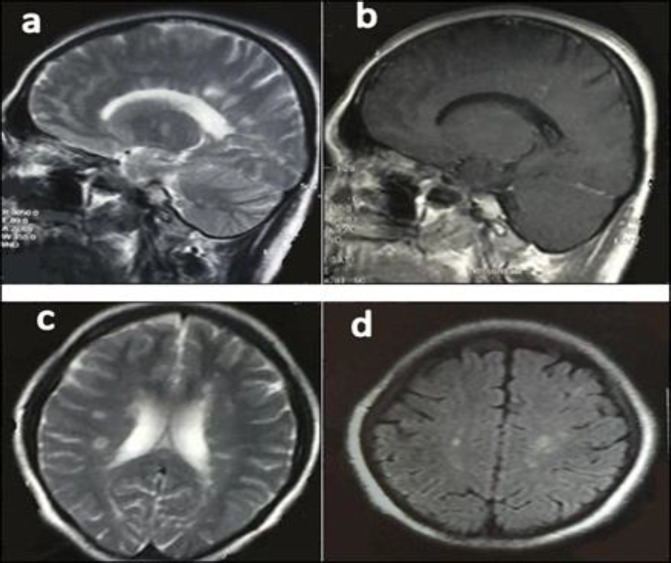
a) Sagittal T2 MRI, multiple hyperintense lesions involving periventricular white matter thalamus and cerebellum; b) Sagittal T1-weighted MRI showed enhancement of one periventricular lesion; c) and d) Axial T2 and FLAIR MRI, respectively; demonstrating multiple periventricular, centrum semioval, and juxtacortical lesions
